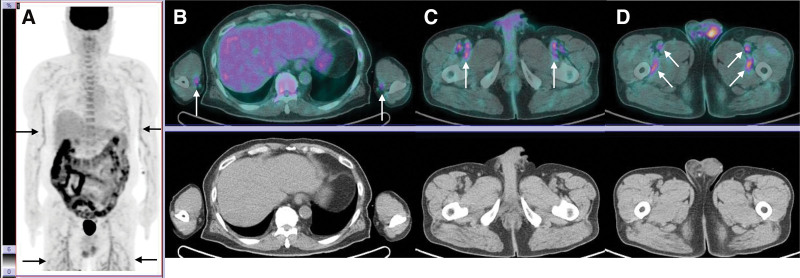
Initial F-18 FDG PET/CT. On the MIP image (A), long and linear hypermetabolism was noted in both arms (arrows), along with branching tree-like patterns of FDG uptake in both thighs (arrows). Multiple tortuous uptakes in the abdomen were physiological uptakes in the bowels. Fusion F-18 FDG PET/CT and CT images (B–D) showed high metabolism in the bilateral brachial and radial arteries, as well as bilateral external iliac, femoral, and perforating arteries (white arrows). These findings are highly suggestive of small- to medium-sized arteritis. CT = computed tomography, FDG = fluorodeoxyglucose, MIP = maximum intensity projection, PET = positron emission tomography.
